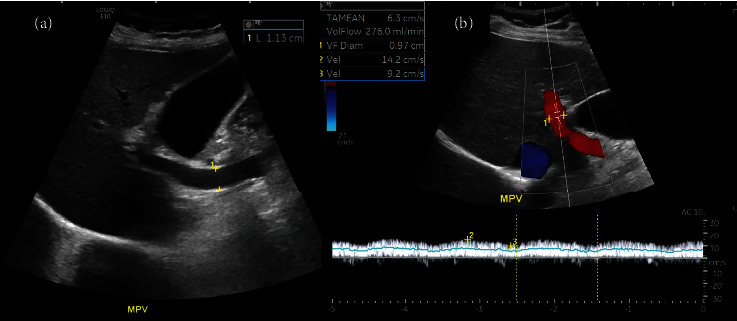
Assessment of the main portal vein: (a) grayscale US measuring a maximal diameter and (b) Doppler US measuring a maximal and minimal velocity and flow volume.
